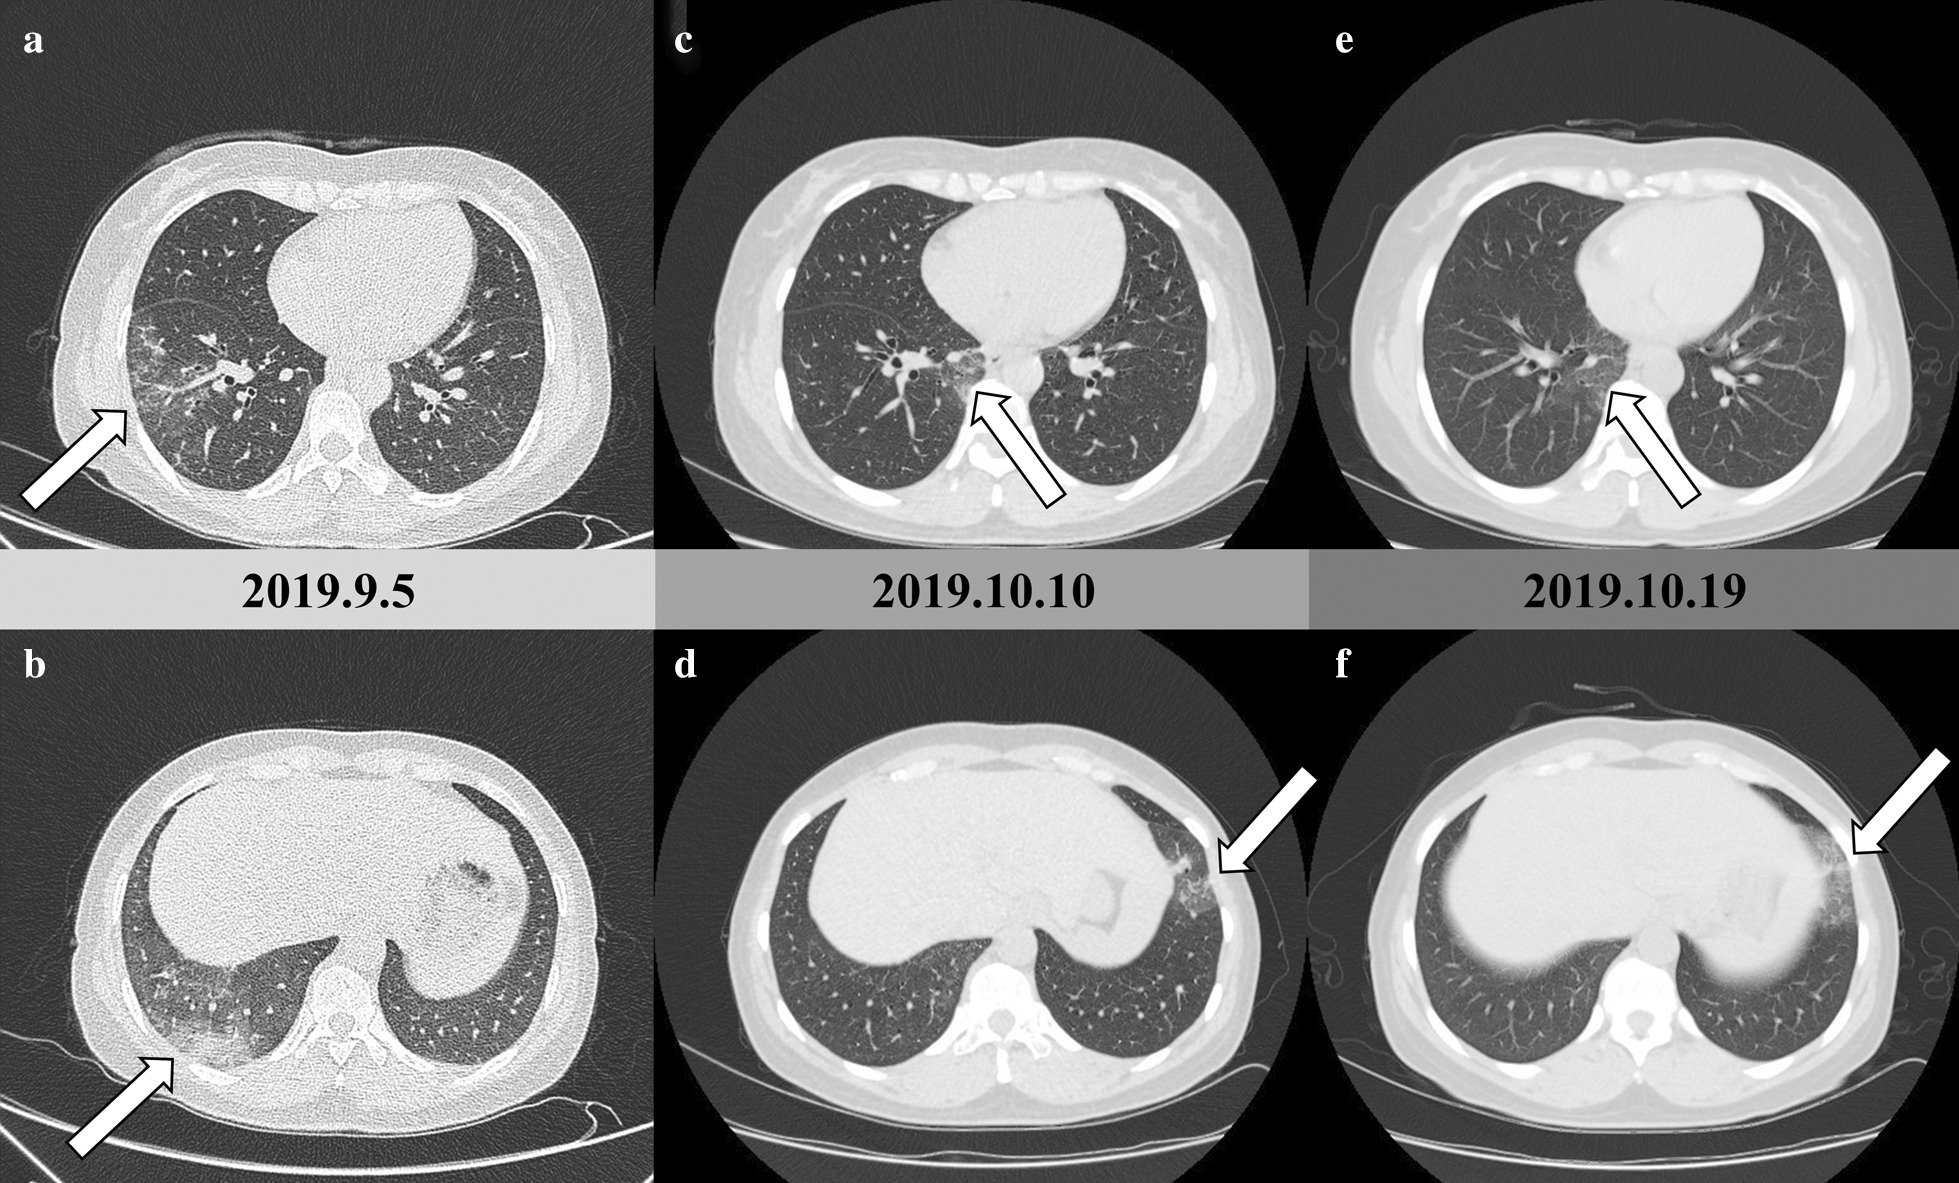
Representative chest CT images showing migratory pulmonary infiltration (white arrows) in a patient with CLPD-NK. a, b CT images captured on September 5, 2019, showing an inflammatory ground-glass opacity change in the lower lobe of the right lung. c, d CT images captured on October 10, 2019, showing the migratory pulmonary shadows with new ground-glass opacities in both of her lungs. e, f CT images captured on October 19, 2019, showing that the bilateral pulmonary ground-glass opacities not only persisted but also displayed an altered distribution
